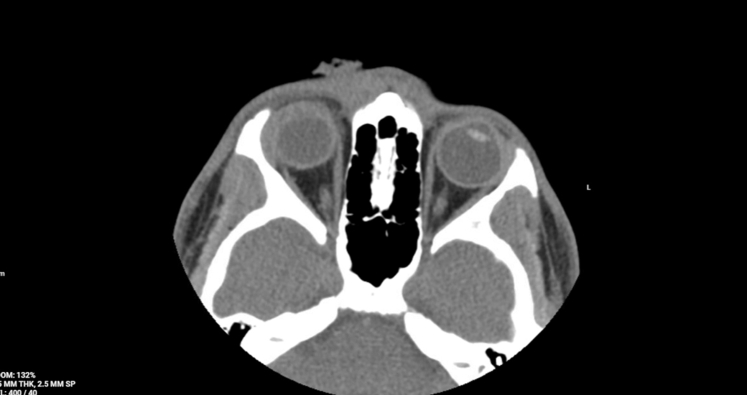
Noncontrast computed tomography scan of the orbits demonstrating absence of the right ocular lens (arrow), a concerning finding in the setting of ocular trauma.
